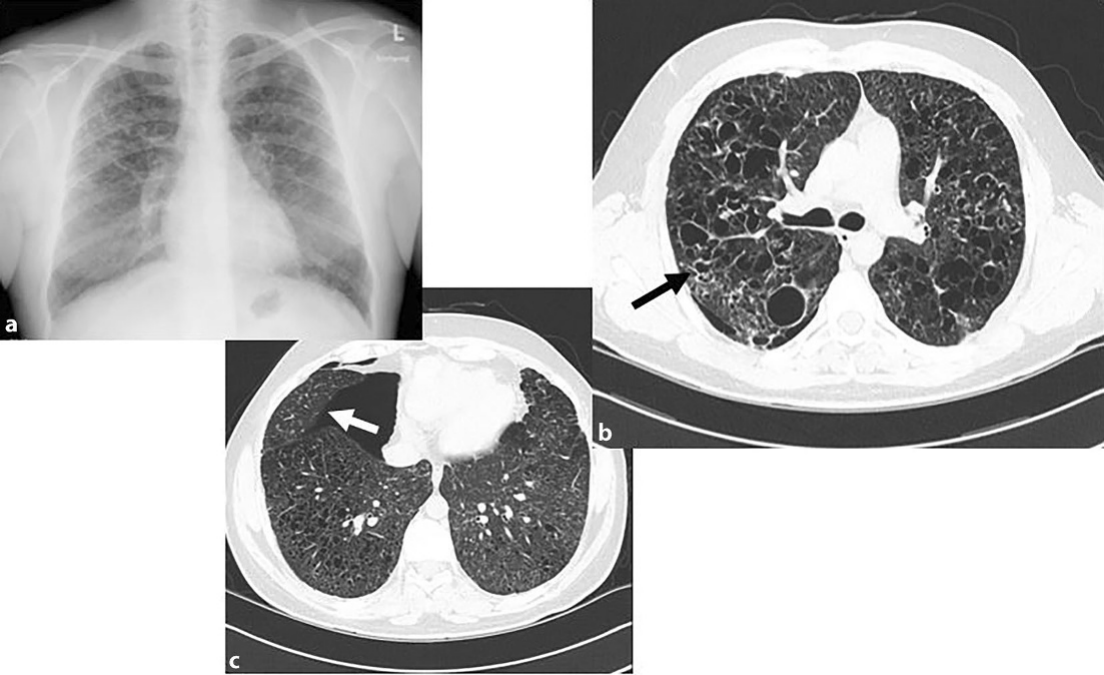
Typisches Bild einer Langerhans-Zell-Histiozytose (LZH). a Posterior-anteriore Röntgenaufnahme mit dem charakteristischen apikobasal betonten Gradienten der zystischen Lungenerkrankung, welche sich im Röntgenbild häufig vorwiegend durch ein führendes retikuläres Muster manifestiert. Die ergänzende CT-Thorax (b) desselben Patienten auf Höhe der Lungenoberlappen zeigt multiple, teils bizarr konfigurierte sowie teils irregulär-wandverdickte Lungenzysten (*schwarzer Pfeil*). Das CT-Verlaufsbild (c) illustriert eine typische Komplikation – die Ausbildung eines Pneumothorax, hier mit einer ausgedehnten Pneumothoraxlamelle rechts (*weißer Pfeil*)
